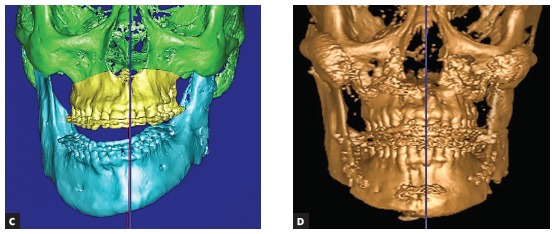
(C-D) Planning of the second-stage bimaxillary osteotomy and actual outcome in CT.
